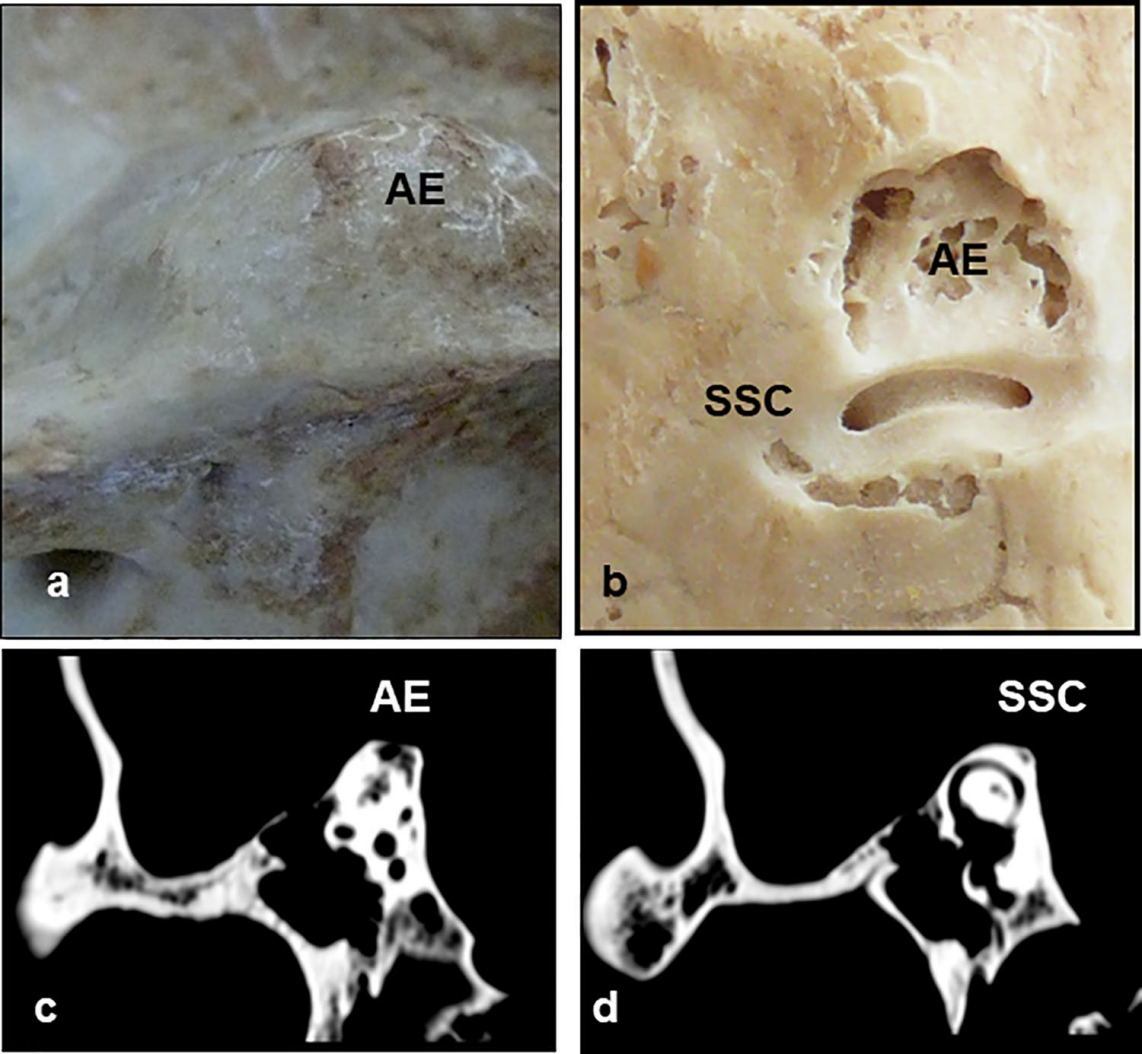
See in a the presence of arcuate eminence prominent type, in b, how the milling of the AE reveals supra-labyrinthine cells and the superior semicircular canal (SSC), remaining anterior and medial to it. Computed Tomography shows how the arcuate eminence corresponds to supra-labyrinthine cells c and in d the superior semi-circular canal. *AE* arcuate eminence, *SSC* superior semi-circular canal
